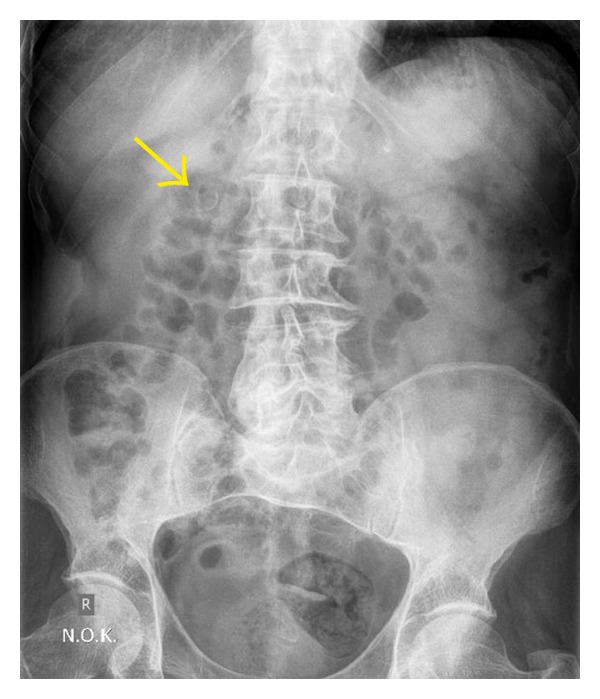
This KUB radiography showed a retroperitoneal curvilinear calcification with discontinuous border, which lied near the right transverse process of the second lumbar vertebra (arrow). This calcification correlated with the ultrasonographically detected pararenal vascular structure with wall calcification.
